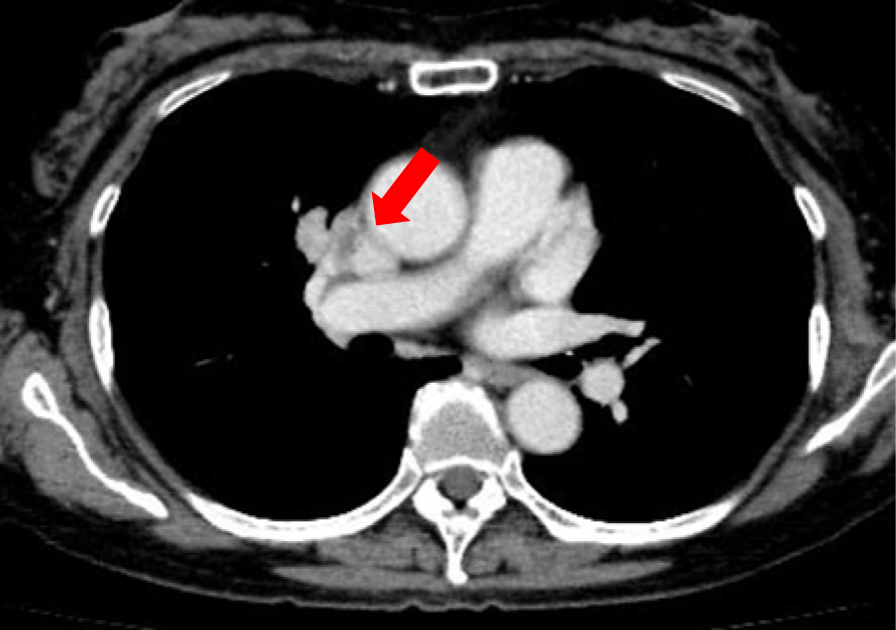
Computed tomography imaging of the lung. Five years after initial surgery, the patient took a routine physical examination including chest computed tomography which revealed an abnormal shadow occupying her right upper lung (*arrow*)
